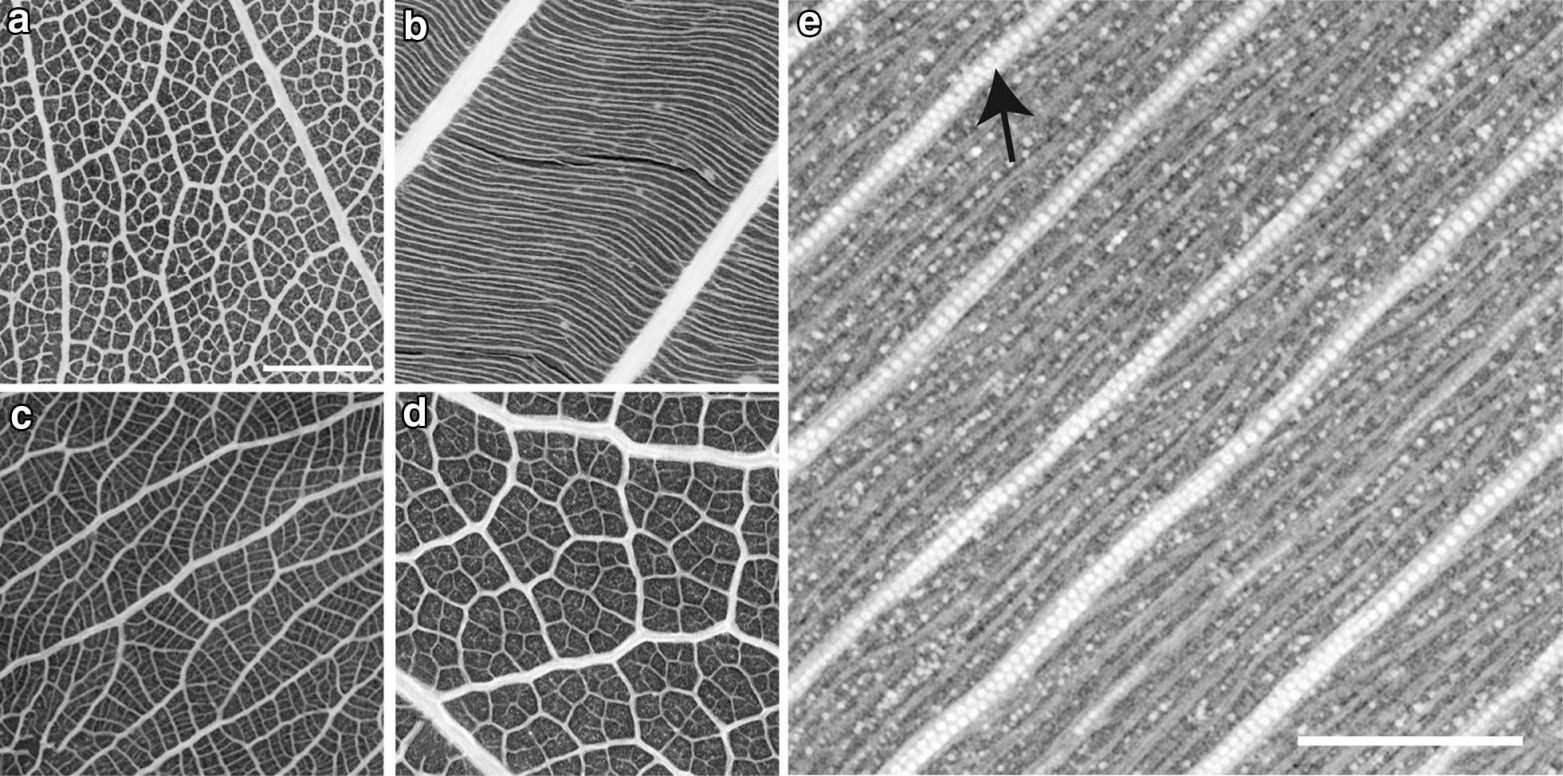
Enlarged sections of very high-resolution imaging (virtual 2D) of fully resolved leaf venation using micro CT. a
